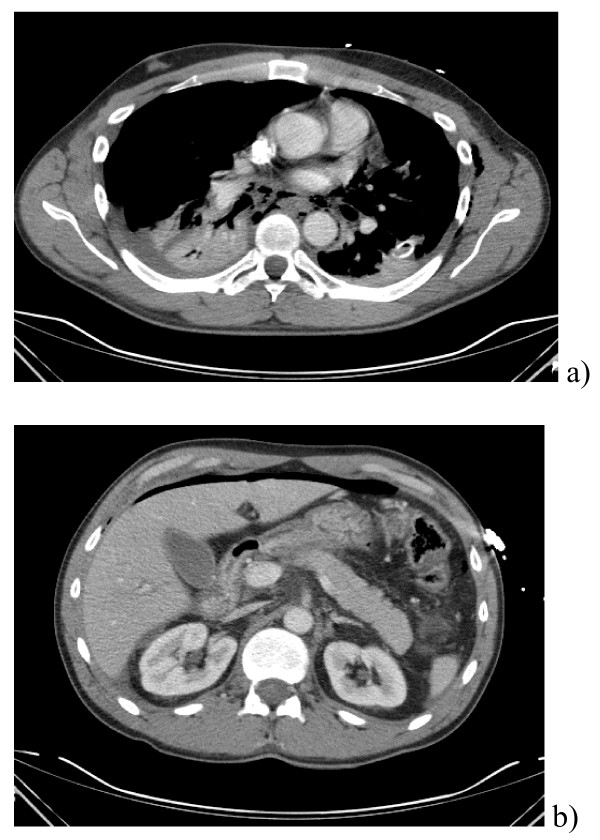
A) CT scan demonstrating pneumomediastinum. B) CT scan of abdomen demonstrating free intra-peritoneal air.
